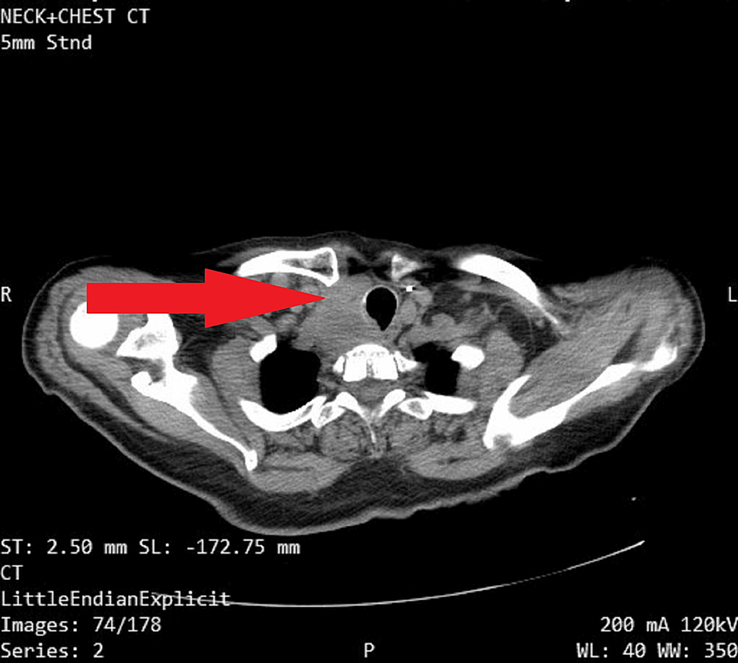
Chest computed tomography (CT) revealed multiple intraparenchymal solid masses in the lower lobe of the right lung, indicating metastasis.
